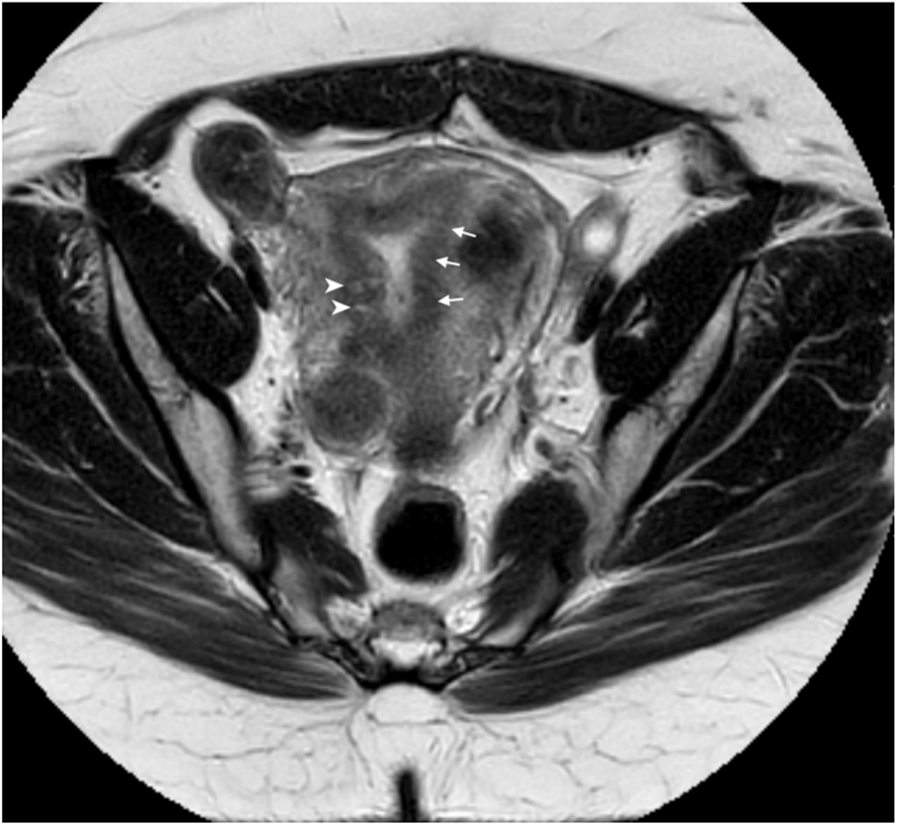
An axial T-1 weighted MRI image shows multiple 1-2 cm myometerial junctional zone cysts (arrowheads) and a thickened junctional zone (arrows)
